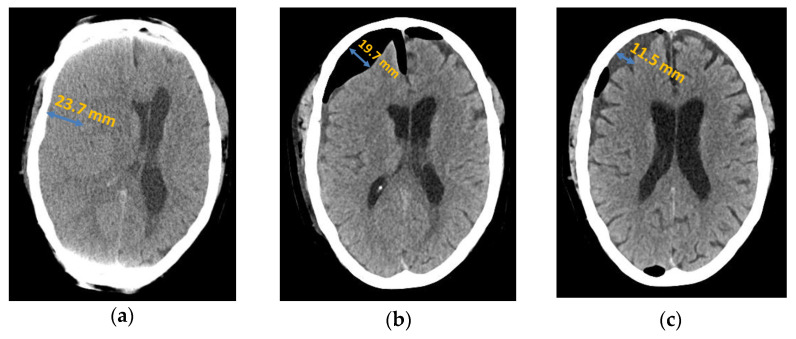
Axial computed tomography of chronic subdural haematoma: (a) preoperative presentation; (b) postoperative subdural air accumulation; (c) recurrence of chronic subdural haematoma.
